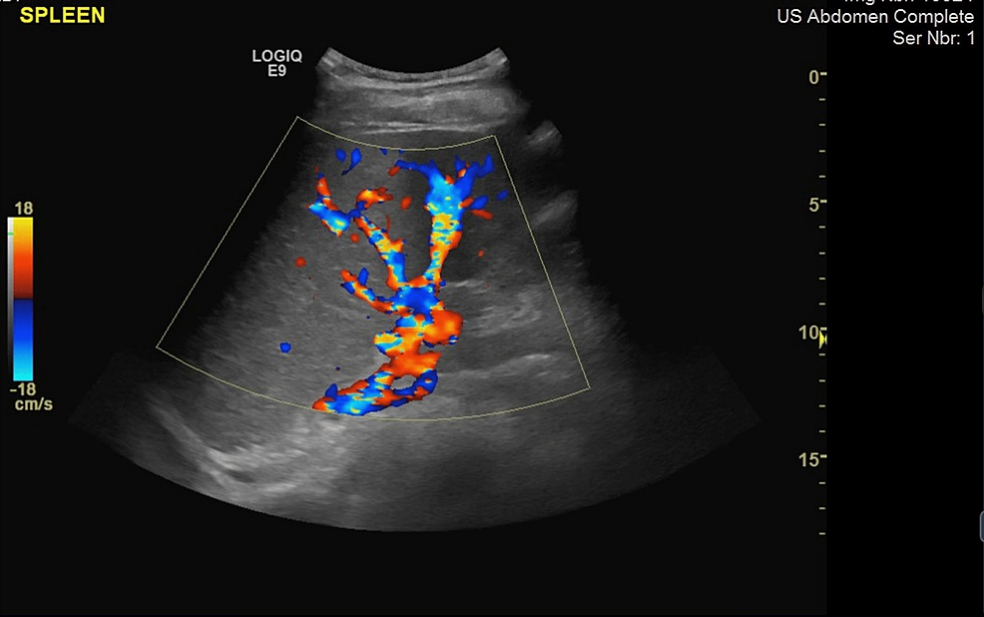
Ultrasound showing a fairly severe splenomegaly without any focal lesions
